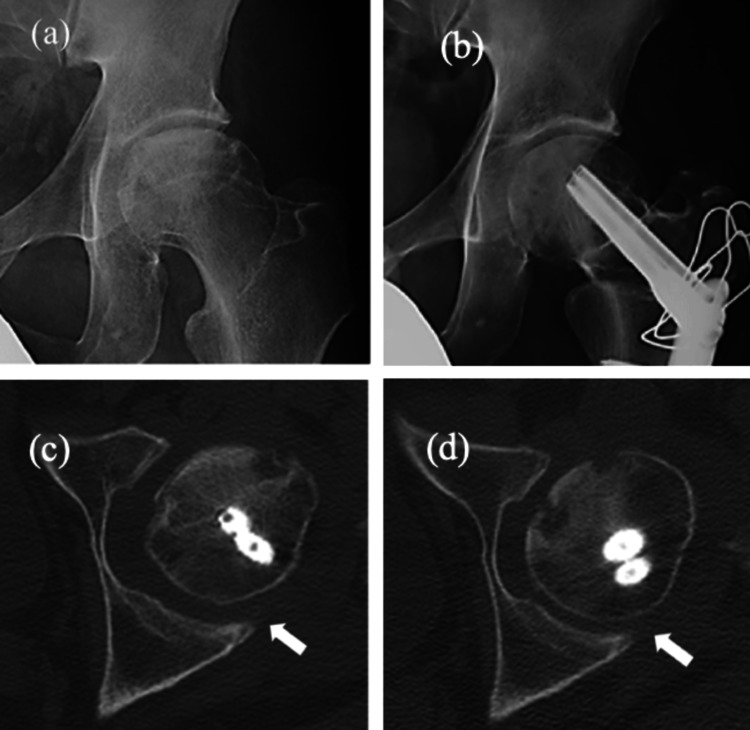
Diagnostic images and CT images of a 37-year-old male. (a) Diagnostic images of a 37-year-old male with alcohol-related osteonecrosis of the femoral head. The pre-operative anteroposterior radiograph of the left hip shows a severely collapsed lesion with no viable area in the loaded portion. (b) A 70º anterior rotational osteotomy with 25º varization was performed. (c) CT images were acquired six weeks post-operatively. The distance from the posterior acetabular joint surface to the posterior joint surface of the femoral head in the neutral position is 6.33 mm (white arrow) on the horizontal CT image. (d) The distance from the posterior acetabular joint surface to the posterior joint surface of the femoral head in the 45° flexion position is 3.81 mm (white arrow) on the horizontal CT image. The translation of the femoral head between the neutral and 45º flexion positions is 2.52 mm, indicating instability. CT: Computed tomography
